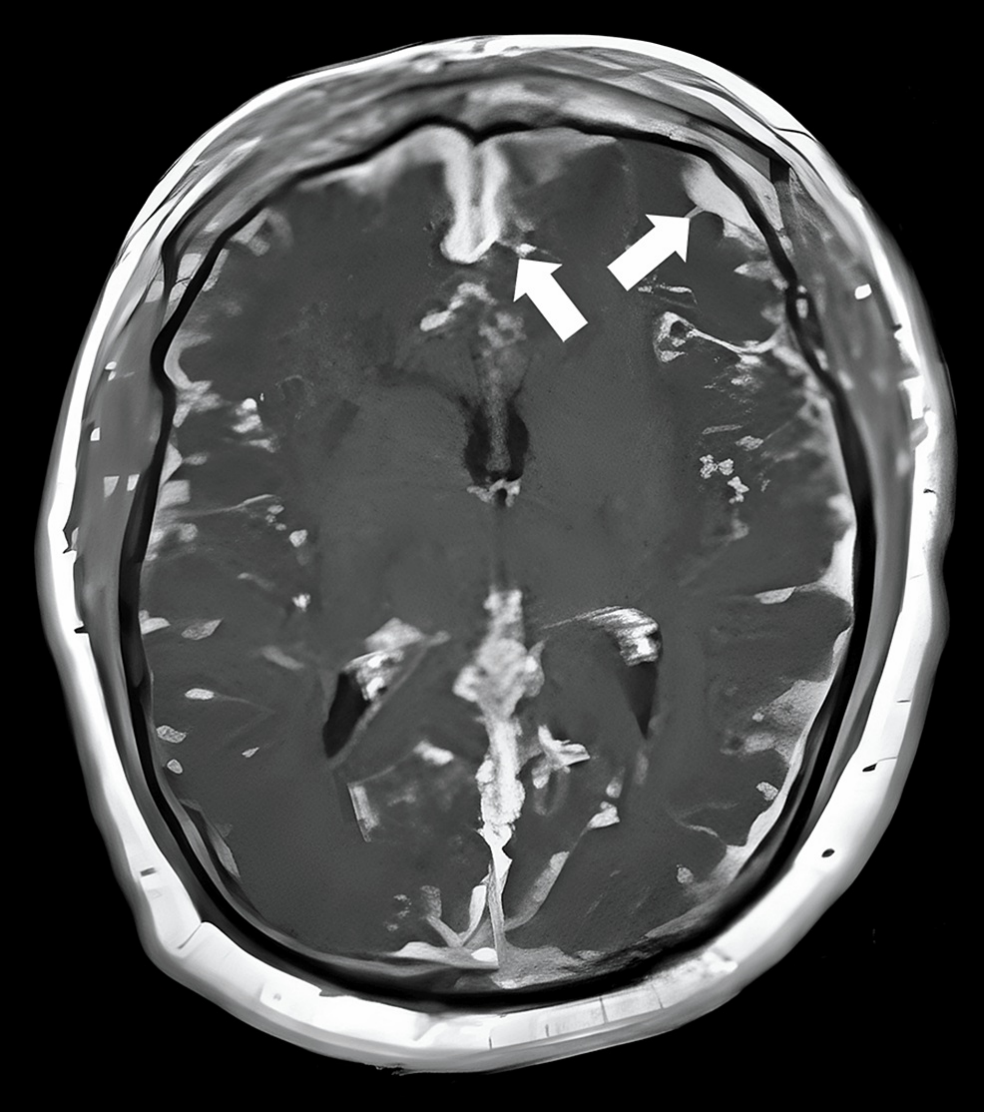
The MRI image elaborating leptomeningeal enhancement, as marked by the arrows.
